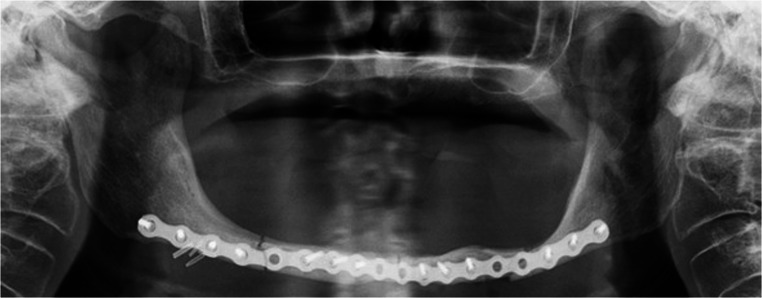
Patient n°1. Postoperative panoramic radiograph showing good reduction of the mandibular fractures
